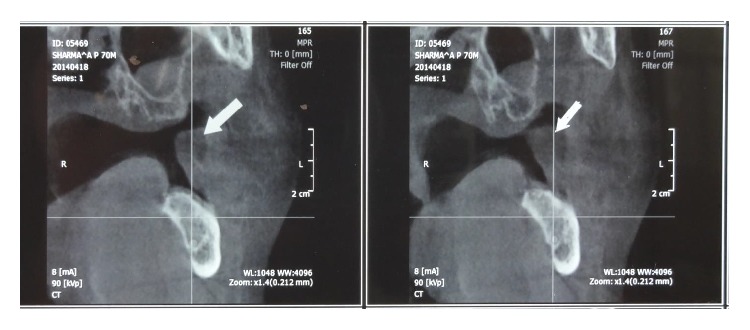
CBCT reveals heterogeneous lesion in left buccomasseteric region extending into left sided retromolar region.
